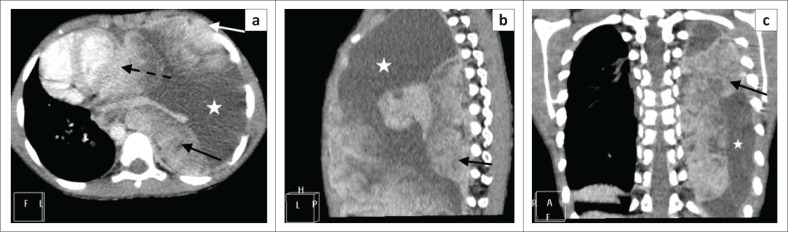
(a–c) Axial, sagittal and coronal contrast-enhanced computed tomography images demonstrate left pleural-based masses which enhance heterogeneously (solid black arrows) with patchy hypodense regions suggestive of necrosis. There is an associated large complex left pleural effusion (white star), atelectasis of the left lung and mediastinal shift to the right. There were no associated intra-tumoural calcifications. Figure 2A – Note the extra-thoracic extension of the mass anteriorly via the intercostal spaces (white arrow) and another pleural-based mass that is inseparable from the pleural pericardial reflection (broken black arrow).
